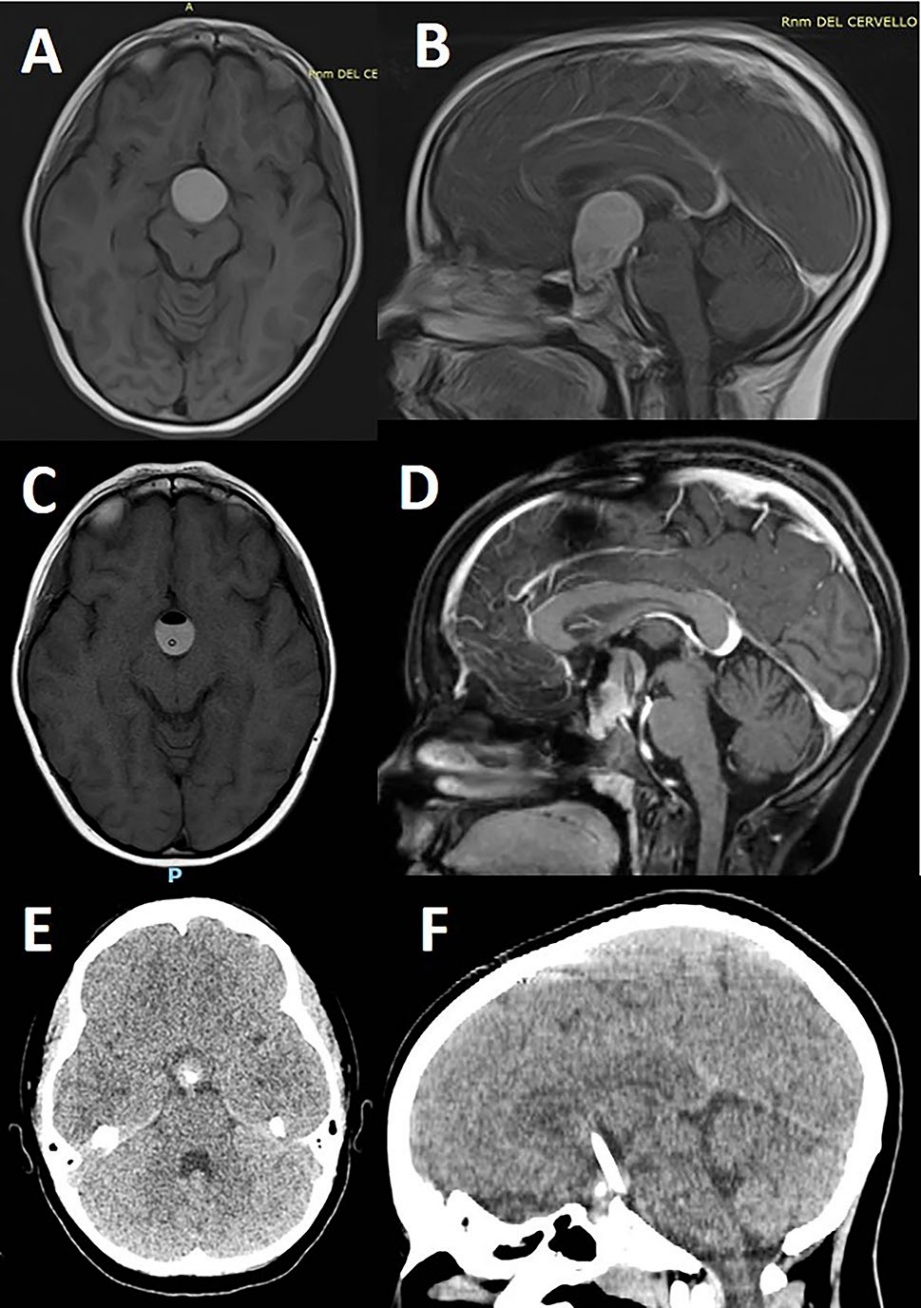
T1 MRI before (A) and after gadolinium administration (B) showing the presence of AC in a 7-year-old girl; C, D the same MRI sequences after navigation-guided placement of intracystic catheter and aspiration of motor-oil fluid, which allowed a significant reduction of the cyst with decompression of the surrounding structures; E, F CT scan performed at the last follow-up (4 years later and after 3 cycles of IA) showing the effacement of the cystic component with evidence of a small, calcified intrasellar, tumor solid portion
